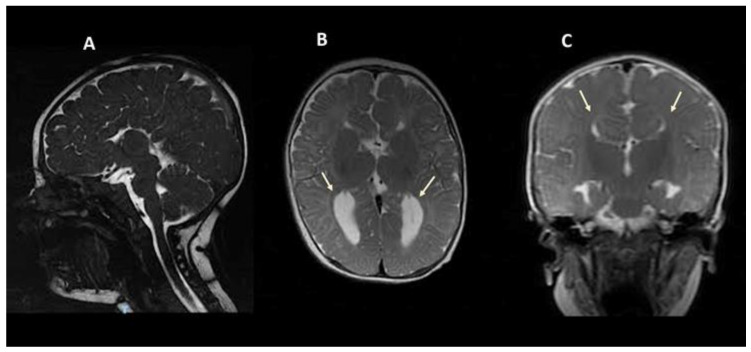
Brain magnetic resonance imaging (MRI) of the proband at age 6 months. Midsagittal FIESTA sequence (A) and axial and coronal T2-weighted sequences (B,C) show complete corpus callosum agenesis (ACC) with associated colpocephaly (B) and Viking helmet appearance of the lateral ventricles (C).
